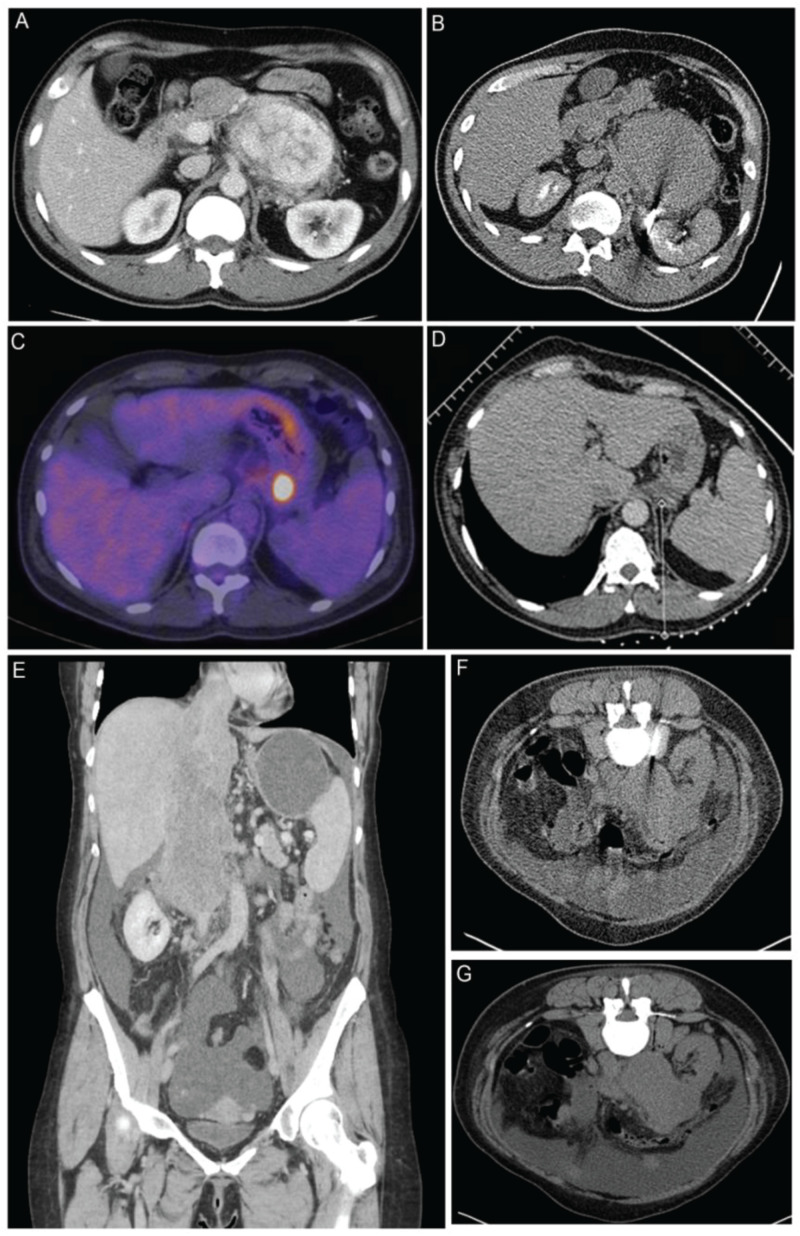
Biopsy examples of patients within the study (A) A computed tomography (CT) of a patient with a highly vascular retroperitoneal mass thought to be too high risk to biopsy at the local hospital. Surgery was also considered to be high risk of R1/R2 resection, and a CT-guided biopsy was recommended by our multidisciplinary team (MDT). (B) Biopsy was performed with a 15-G/16-G coaxial needle. The tract was plugged with 16-G Hunter plugs, and there were no complications. The biopsy showed an inflammatory myofibroblastic tumor, which responded well on steroids and an operation was avoided. (C) PET/CT of a 57-year-old patient with relapsed Hodgkin’s lymphoma after six cycles of ABVD chemotherapy. There was response in all sites of disease with the exception of a plaque of tissue behind the fundus of the stomach, which appear [18F]-fluorodeoxyglucose (FDG) avid on PET scan. A decision of the MDT was made to biopsy the lesion in order to exclude transformation of lymphoma. (D) The 17-G coaxial needle was placed medial to the left adrenal and above the splenic vessels adjacent to the lesion. Three cores were taken, and the tract was plugged. There were no complications. The biopsy showed Hodgkin’s lymphoma, which responded well to systemic therapy and consolidation RT. (E) Coronal CT images of a 48-year-old patient with a large tumor of the inferior vena cava (IVC) extending from the level of the renal veins to the right atrium. Occluded hepatic veins and ascites can be seen on the scan. (F) The lesion was biopsied with a 15-G/16-G coaxial needle. (G) The tract was plugged with three gelfoam pledgets. A diagnosis of leiomyosarcoma of the IVC was made, and the procedure had no complications.
